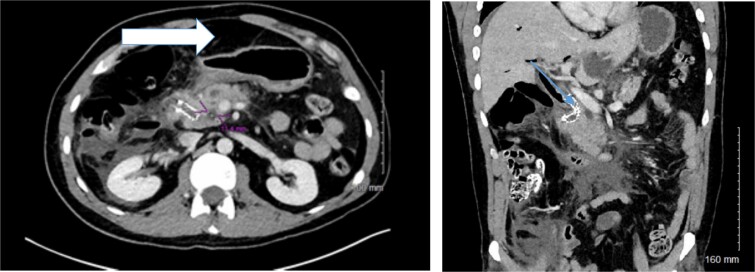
Preoperative and intraoperative findings of duodenal perforation and reconstruction. Preoperative CT- scan of the abdomen delineating the amount of free intra-peritoneal air (arrow) and the location of the biliary metallic stent (arrow).
